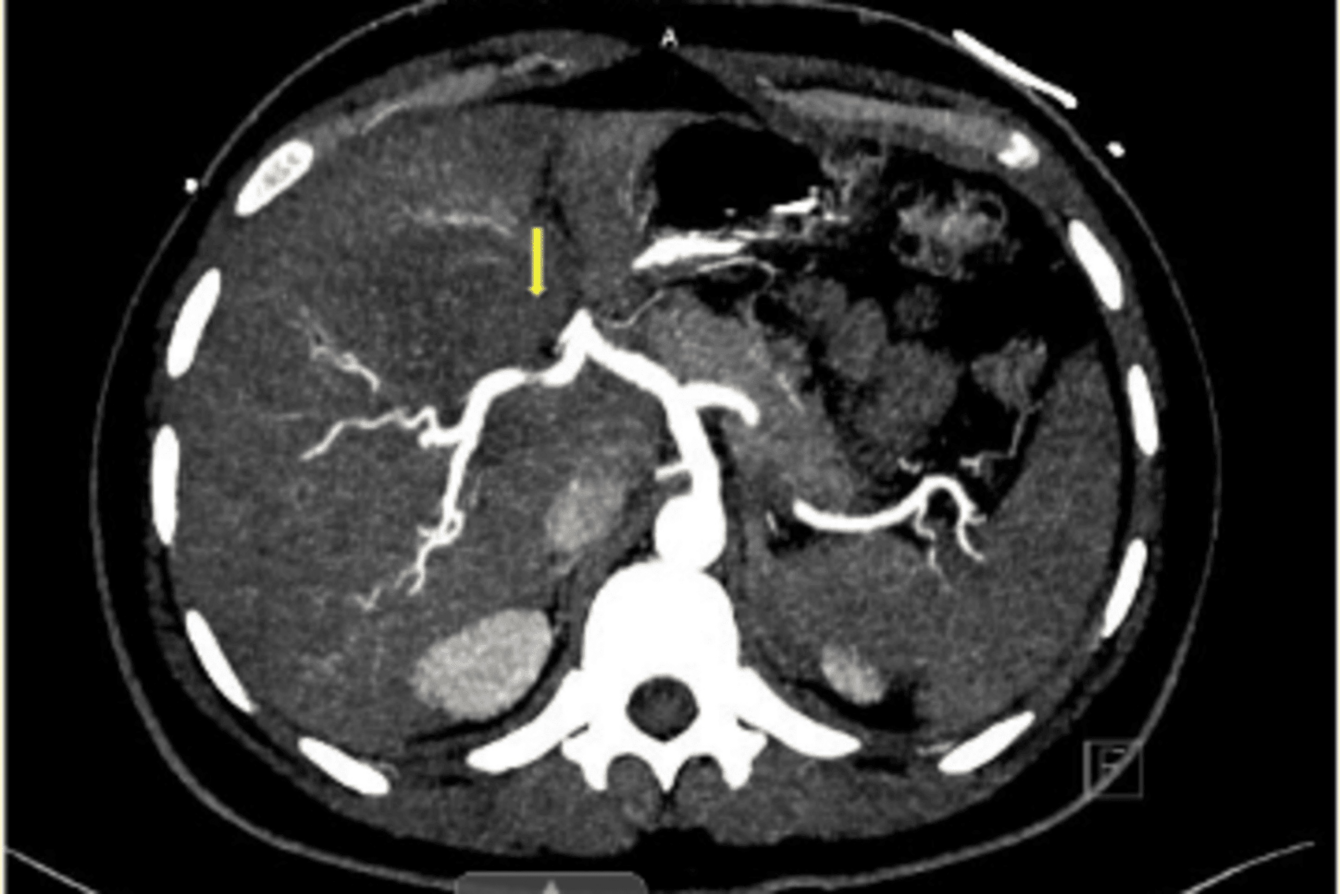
CT angiography of abdomen/pelvis showing thrombosis of the portal vein (yellow arrow)
